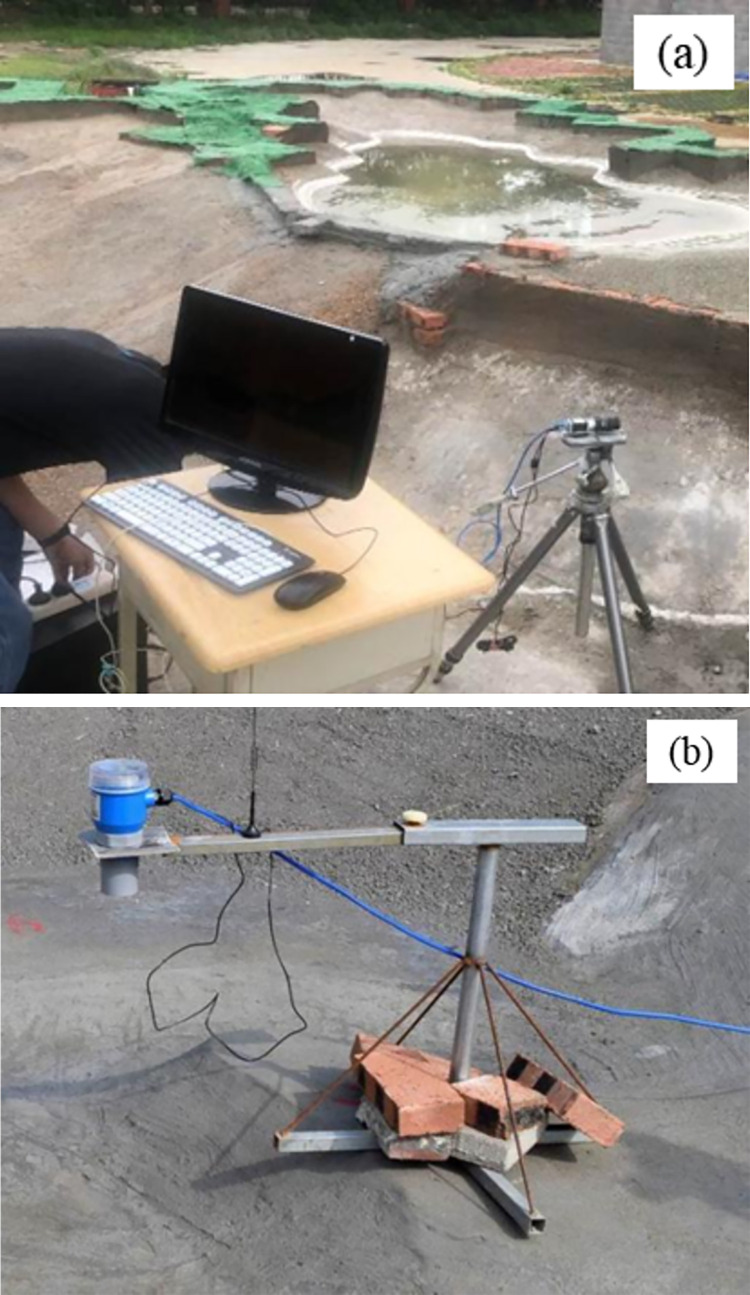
Test equipment: (a) HD camera monitoring system; (b) Ultrasonic liquid level meter.
